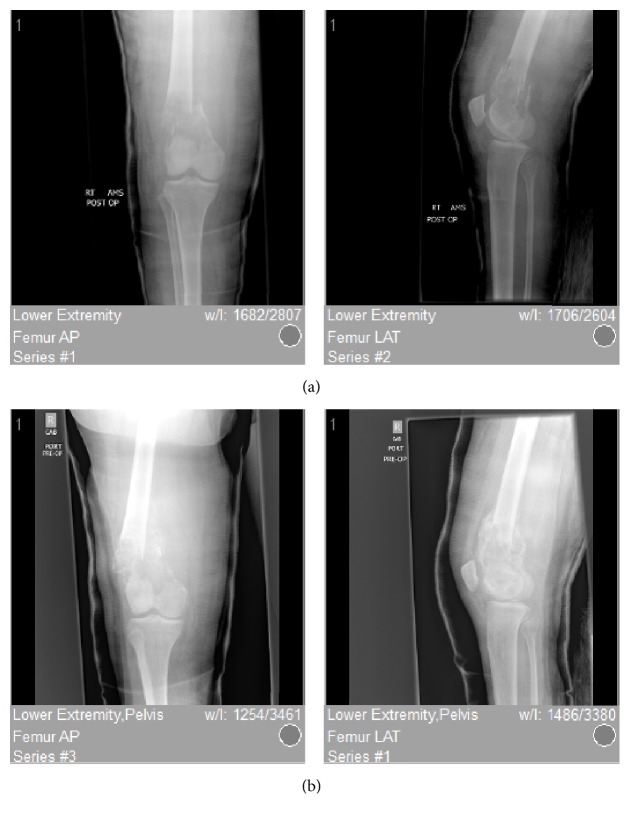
Preoperative AP/lateral radiographs showing an expansile, aggressive-appearing, lytic lesion of the distal femur with associated extra-articular pathologic fracture. (a) shows prereduction AP and lateral radiographs of a left distal femur pathologic fracture; (b) shows AP and lateral radiographs status, after reduction and splinting.
